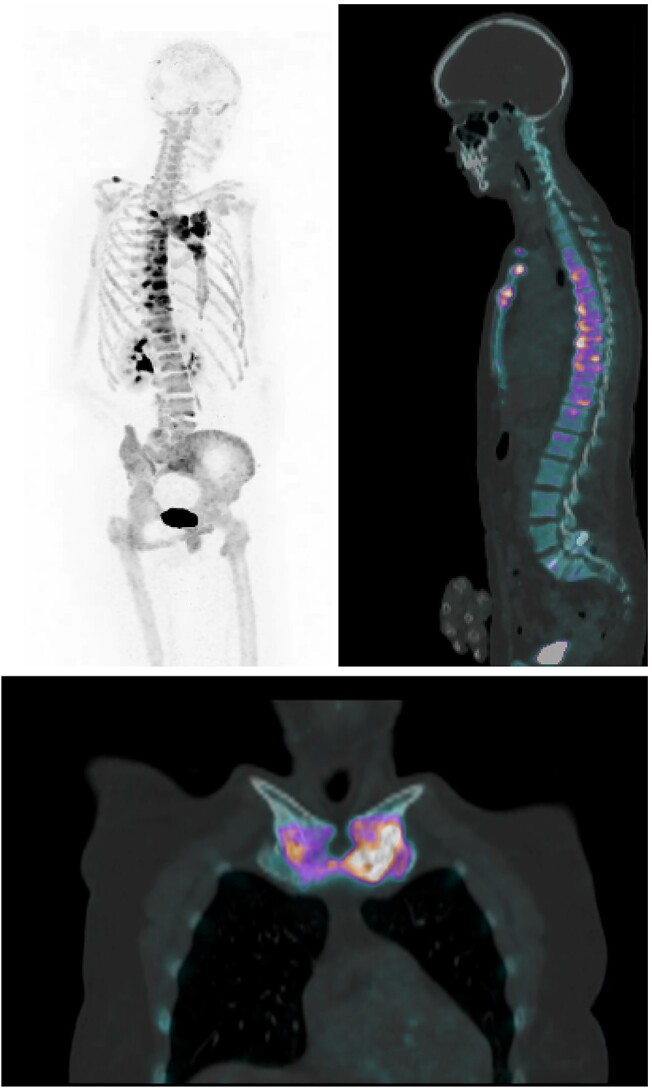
Maximum intensity project (MIP; left upper panel), sagittal (right upper panel), and coronal (lower pane;) views of CNO on [^18^F]NaF-PET/CT in a patient with involvement of the anterior chest wall and thoracic vertebrae. Abbreviations: CNO, chronic nonbacterial osteitis; [18F]NaF-PET/CT, sodium fluoride-18 positron emission tomography/computed tomography.
